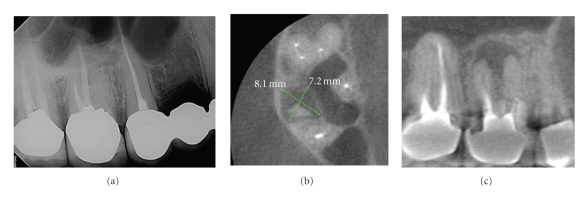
This patient was referred for discomfort and swelling in the maxillary right quadrant. While an initial periapical radiograph (a) clearly demonstrated an untreated mesial root in the maxillary first molar, periradicular periodontitis was undetectable. CBCT images ((a) axial, (b) sagittal) clearly identify a large apical radiolucent lesion associated with the mesial root extending to the distobuccal root. The tooth was retreated and the symptoms subsided.
